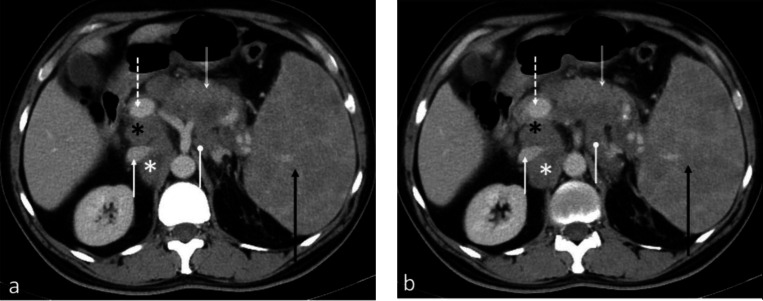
Contrast enhanced axial CT abdomen of a 46-year-old female with lymphoma shows enlarged retrocaval (white asterisk) and portacaval (black asterisk) lymph nodes. Portal vein and IVC are indicated by dotted and solid white arrows respectively. Also noted are an enlarged celiac lymph node (oval arrow) and spleen (black arrow). Heterogenous enhancement of the pancreas (double arrow) is suspicious of lymphomatous involvement. Image b is caudal to the image a. There is little consensus in literature on the best single measurement for assessing size of the spleen as its shape and configuration makes linear measurement difficult. The upper limit of normal adult splenic length is traditionally considered to be 12 cm
